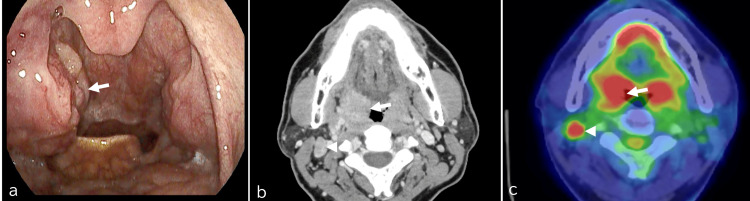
Endoscopic findings of the oropharynx and neck imaging in a case of oropharyngeal cancer. a.The laryngoscopic findings revealed enlargement of the right tonsil (arrow), b. Contrast-enhanced CT imaging demonstrated hypertrophy of the right tonsil (arrow) along with swelling of the cervical lymph node (arrowhead), c. PET-CT showed significant FDG uptake in the right tonsil (arrow) and cervical lymph node (arrowhead), indicating hypermetabolic activity consistent with malignancy.
